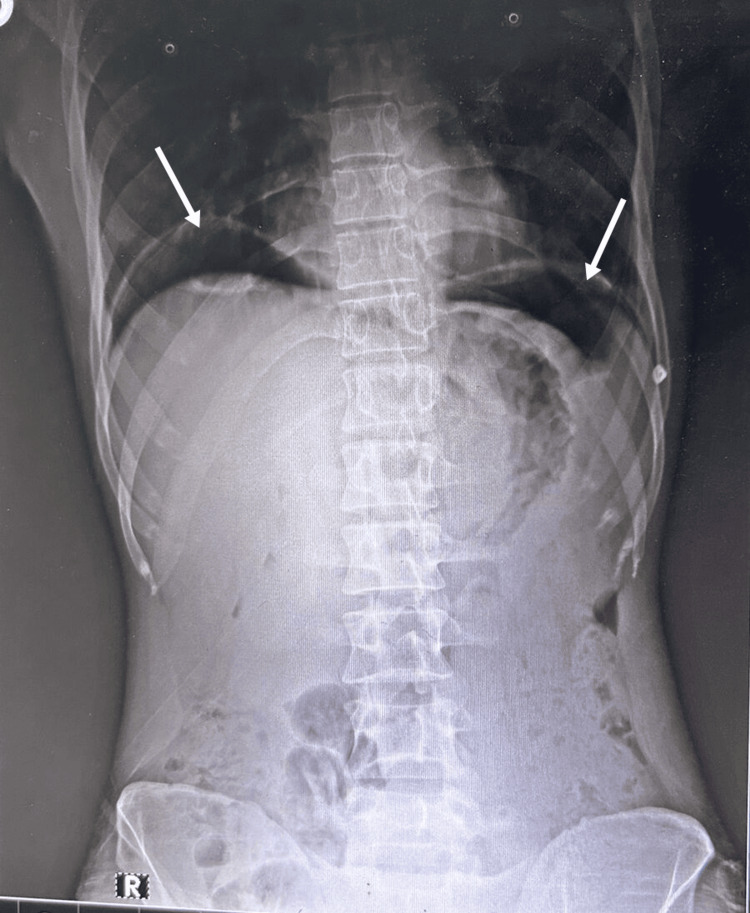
Pneumoperitoneum on abdominal X-ray (erect) performed 72 hours after the admission. White arrows show air under both domes of the diaphragm.
